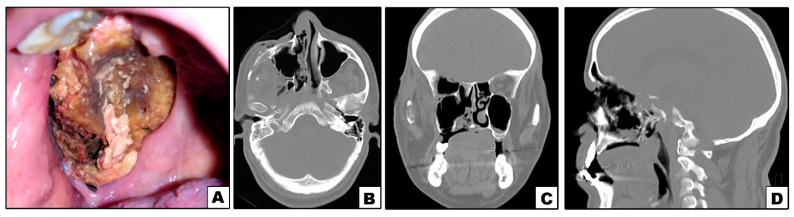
Case#5: (A) Exposed necrotic palatal and alveolar bone on right side. (B–D) Axial, coronal and sagittal CT showing bone destruction involving right maxilla and orbit.
